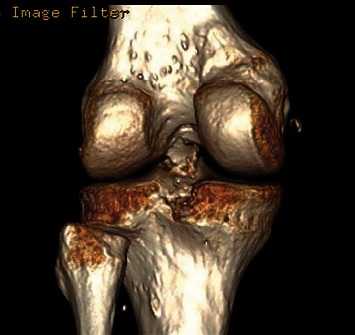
CT scan of Case 2. The 3D reconstruction shows the avulsed tibial fragment from the posterior tibial plateau.
